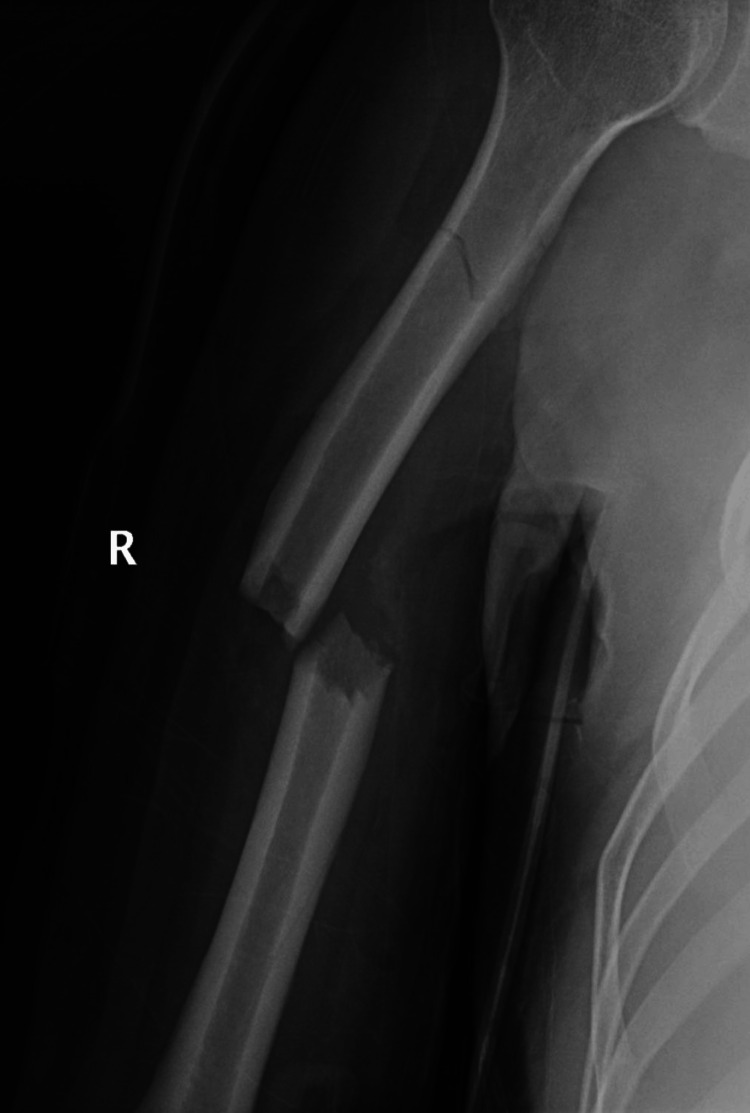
Plain radiograph shows a right humerus fracture callus starts to appears 11 days after the accident
